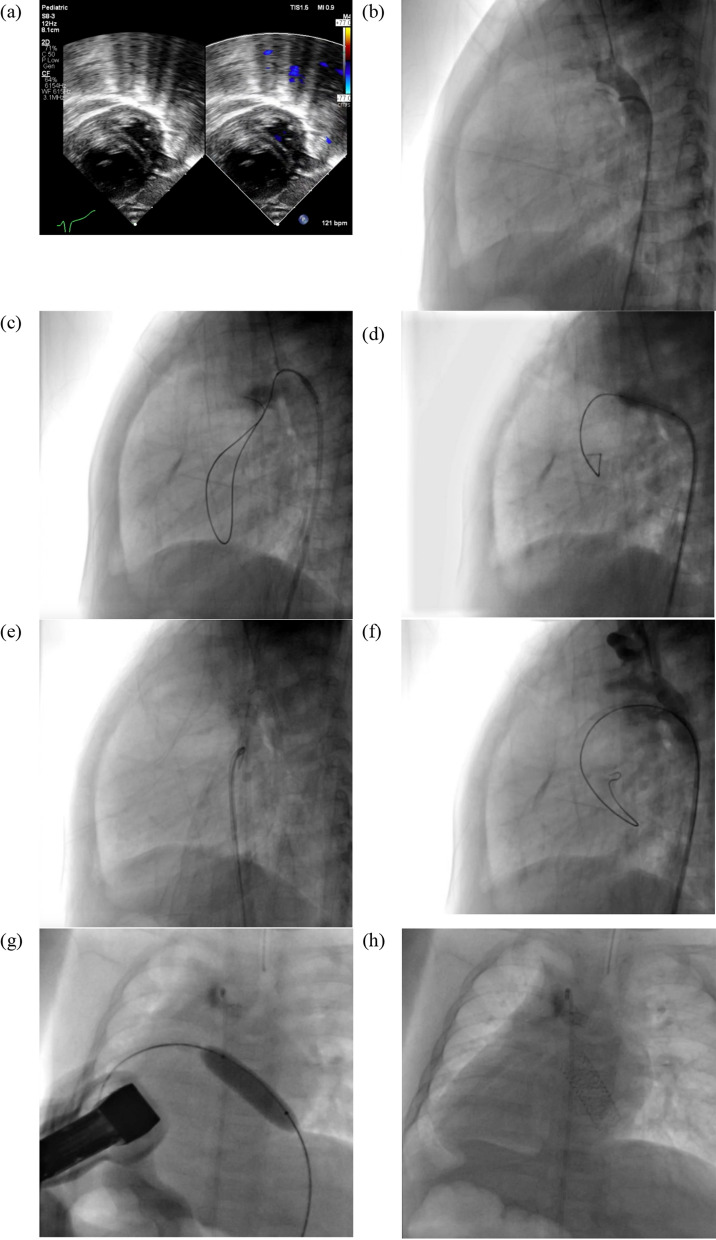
Ductal Recanalization and IAS Stenting in d-TGA/IVS. (a) Echocardiography showed an LVMI of 36–41 g/m2 and a D-shaped left ventricle, indicating higher right ventricular pressure. (b) Angiography revealed a completely occluded PDA with no pulmonary artery flow. (c) A V-18″ wire was successfully advanced into the right pulmonary artery. (d) A 3.0 × 15 mm Emerge balloon was inflated at 12 atm for 5 s. (e) A 4.0 × 16 mm Promus Premier stent was deployed at 16 atm, flared at 18 atm. (f) Post-procedure angiography confirmed proper stent placement with restored flow. (g, h) A 10.0 × 29 mm Omnilink Elite stent was deployed in the interatrial septum at 14 atm for 5 s
